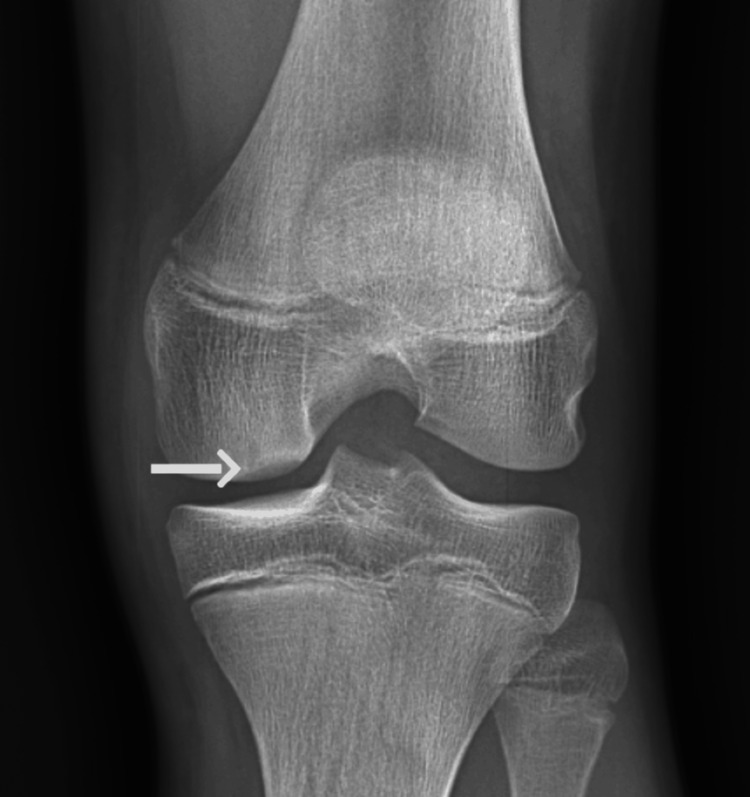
X-ray of the left knee demonstrating tunnel view. The white arrow indicates a subchondral defect at the medial femoral condyle of the left knee.
